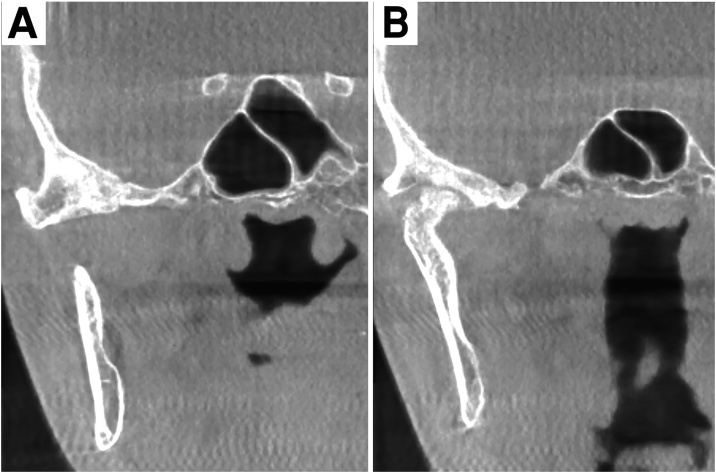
Follow up CBCT one year postoperatively. (A) Relative hypodensity of the previous surgical site in the center of endosteal sclerosis involving the surrounding bones of the skull base. (B) The residual surgical defect in glenoid fossa.
